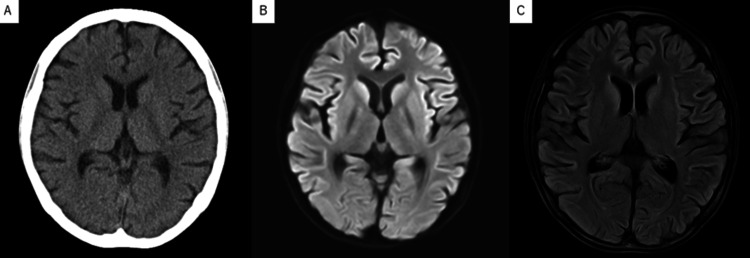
Radiologic findings of the brain (A) The computed tomography showing the generalized brain atrophy. (B) The magnetic resonance imaging (diffusion-weighted imaging) showed no findings, such as Wernicke encephalopathy. (C) The magnetic resonance imaging (fluid attenuated inversion recovery) showing the generalized brain atrophy.
